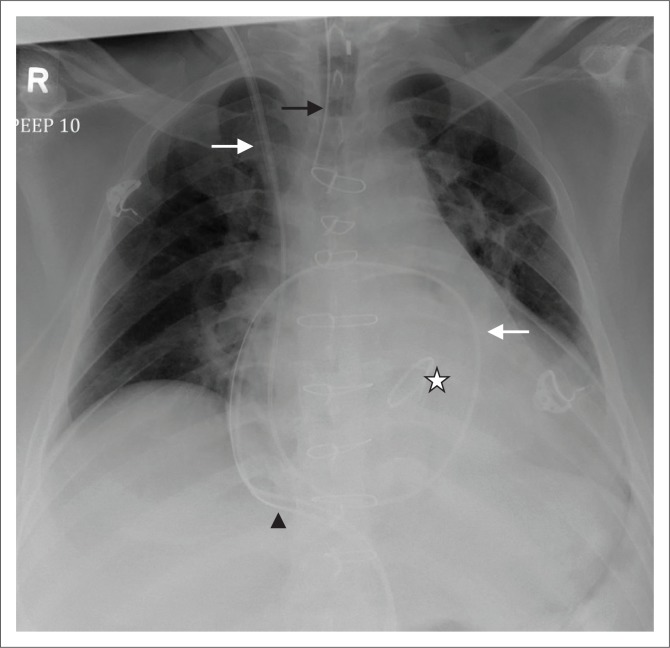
Chest radiograph showing a Swan Ganz or pulmonary artery catheter (white arrow), with its tip in the right pulmonary artery. The other devices on this chest radiograph are an endotracheal tube (black arrow), a mediastinal drainage tube (arrow head) and a prosthetic aortic valve (star).
